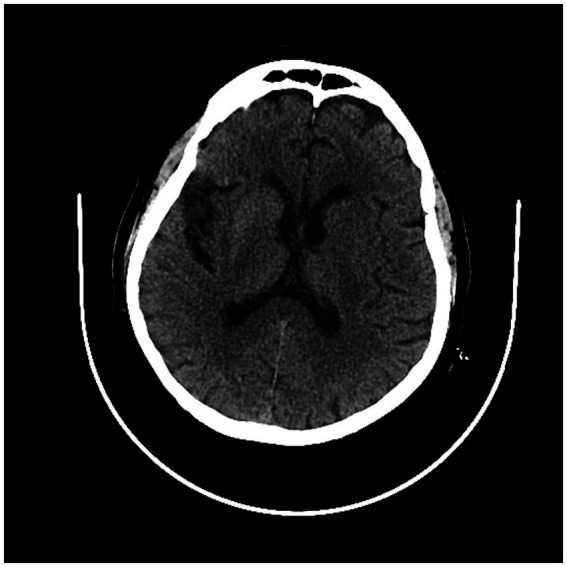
CT scan showing right total insular cortex (TIC) ischemic lesion.
